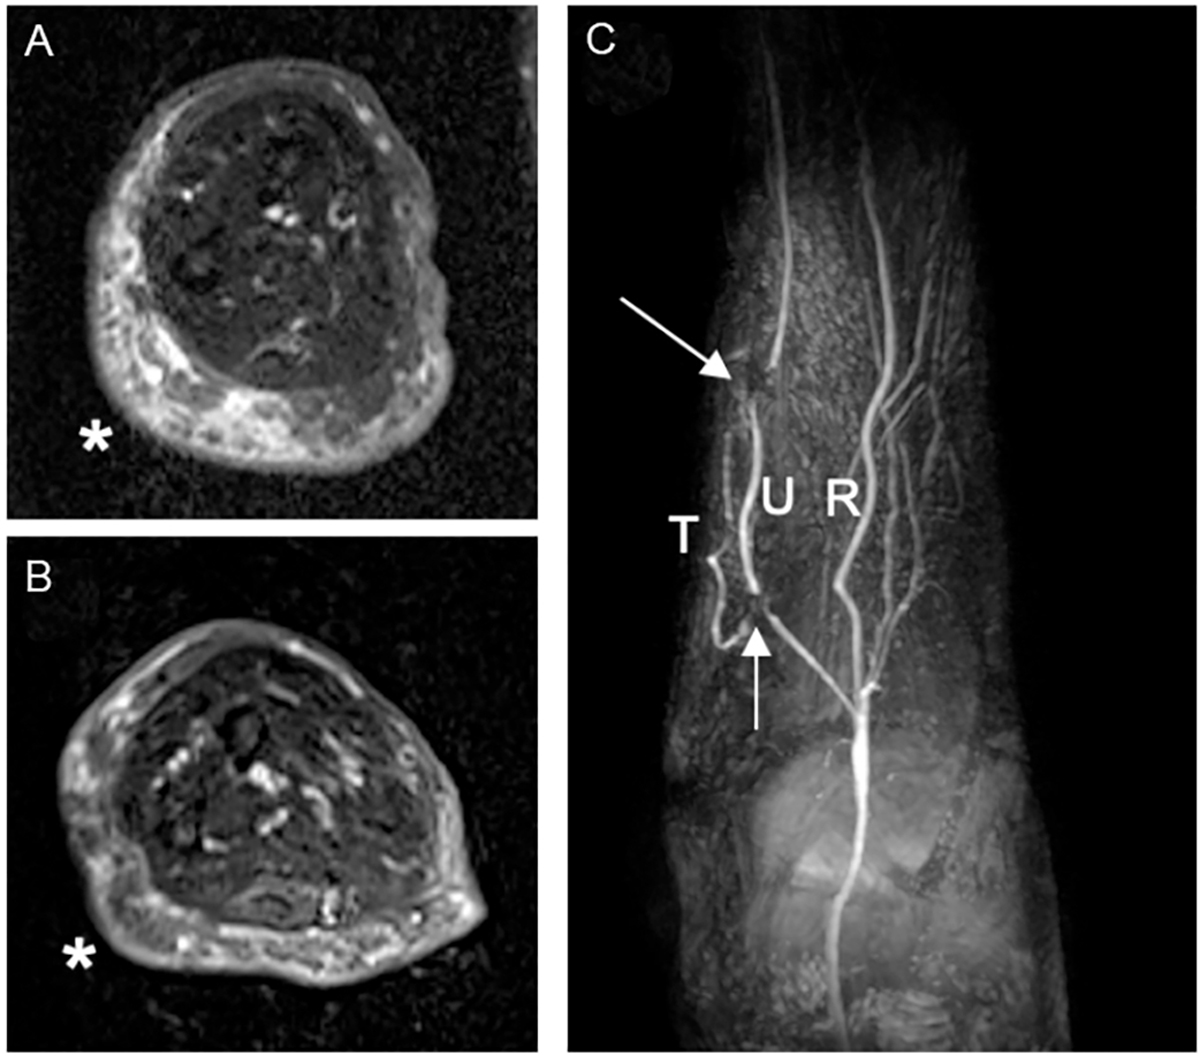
Improvement of upper extremity edema following a vascularized lymph node transplant. Axial fat-suppressed T2-weighted images across the mid right forearm in a patient with right upper extremity lymphedema, pre- (A) and 1-year post-transplant (B) demonstrating interval marked decrease in subcutaneous edema and thickening along the ulnar aspect (*). Arterial-phase post-contrast maximum intensity projection image (C) shows patent flow-through omental artery of the transplant (T). The arrows show signal voids from surgical clips demarcating anastomoses to the ulnar artery (U). R: Radial artery.
